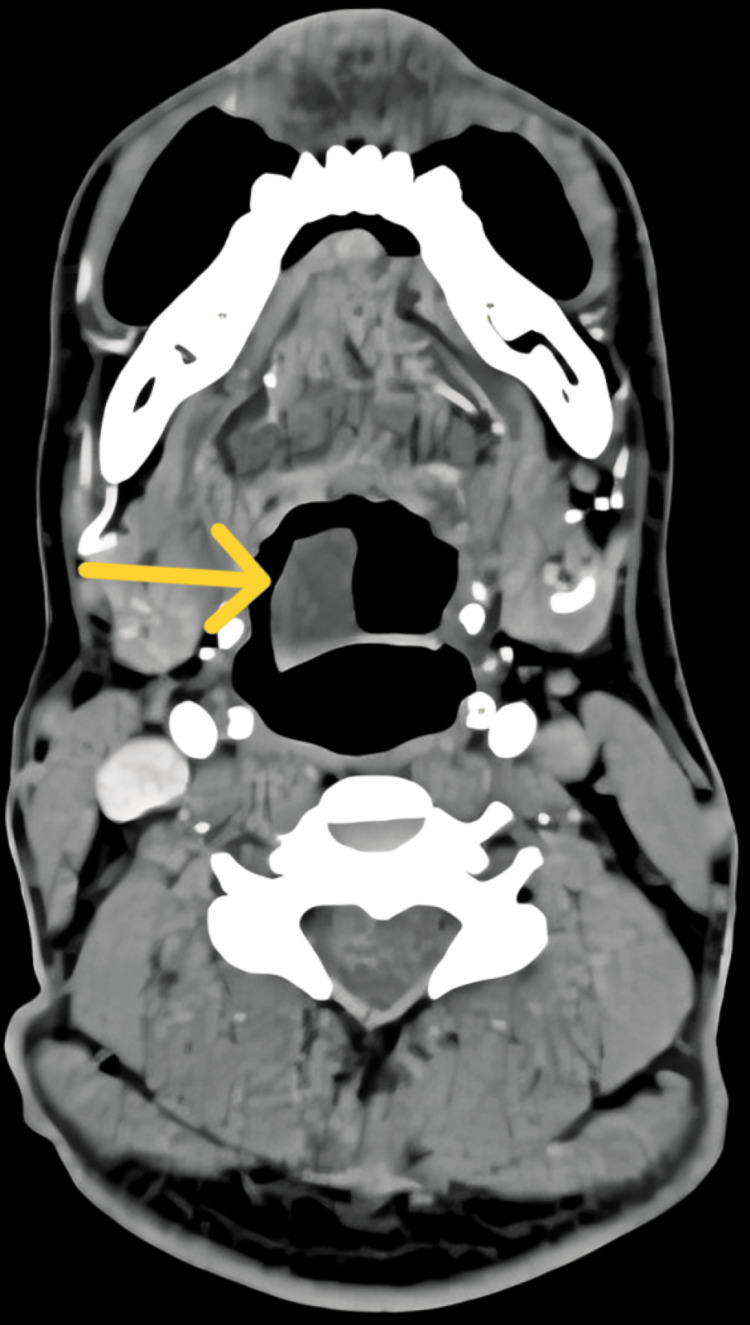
Computed tomography of the neck, where the axial cuts show the cyst arising from vallecula (yellow arrow)
